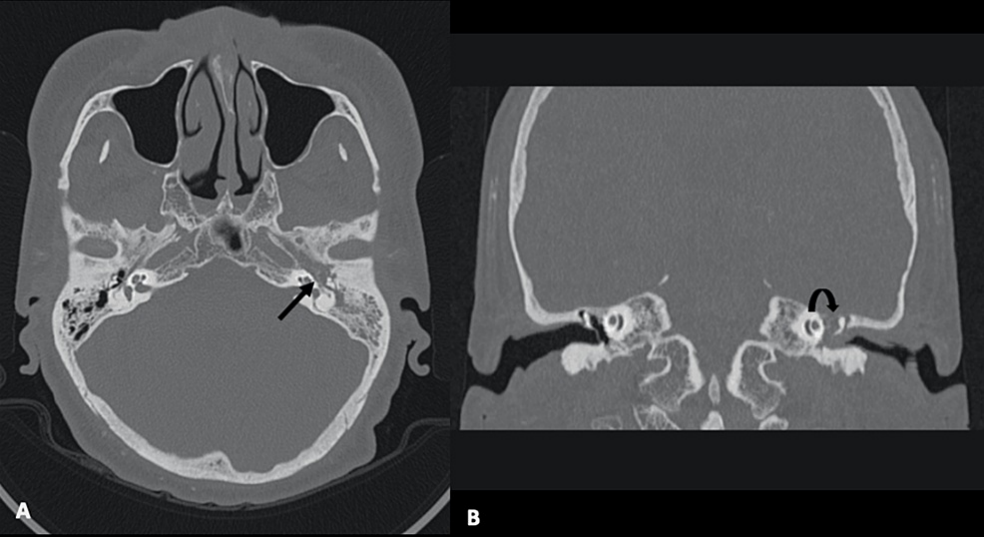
HRCT temporal in (A) axial and (B) coronal views taken about one month apart, demonstrating erosion of the middle ear cavity (long arrow) and worsening dehiscence of the left tegmen tympani HRCT: high-resolution computed tomography
